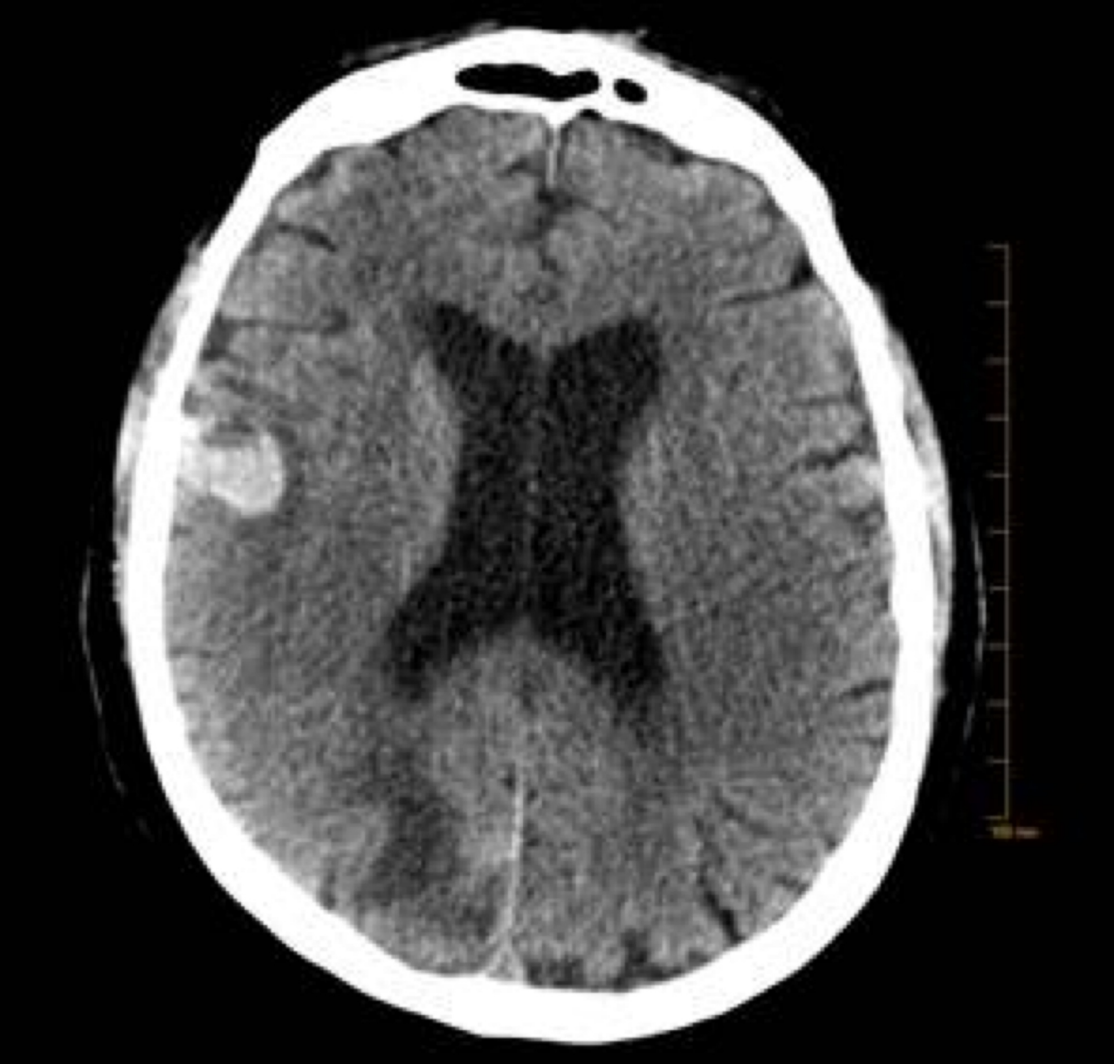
CT brain without contrast demonstrating a rounded area of hemorrhage with hyperdensity measuring 1.8 cm involving the right frontal cortex just above the Sylvian fissure with surrounding slight vasogenic edema CT: computed tomography
